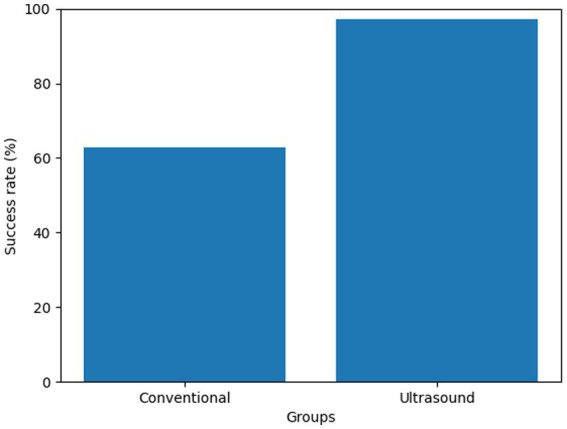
First-attempt success rate of double-lumen tube placement. Ultrasound guidance significantly improved success rate compared with the conventional method (*p* = 0.001).
